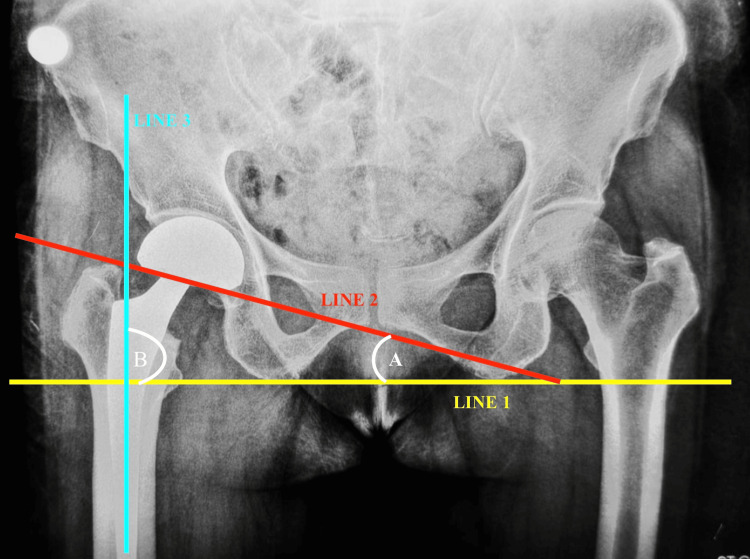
Neutral AP pelvic radiograph demonstrating reference lines and angular measurements (A and B) Line 1: a line drawn tangential to the most inferior aspects of the ischial tuberosities, which was used as a reference line. Line 2: Drawn along the inferior margin of the acetabular component, and Line 3: Drawn along the center of the long axis of the femoral stem. Angle A is defined as the point where the reference line intersects the line drawn from the inferior border of the acetabular component (line of ischial tuberosities). The ischial reference line and a line drawn across the middle of the long axis of the femoral stem intersect to generate angle B. AP, anteroposterior
